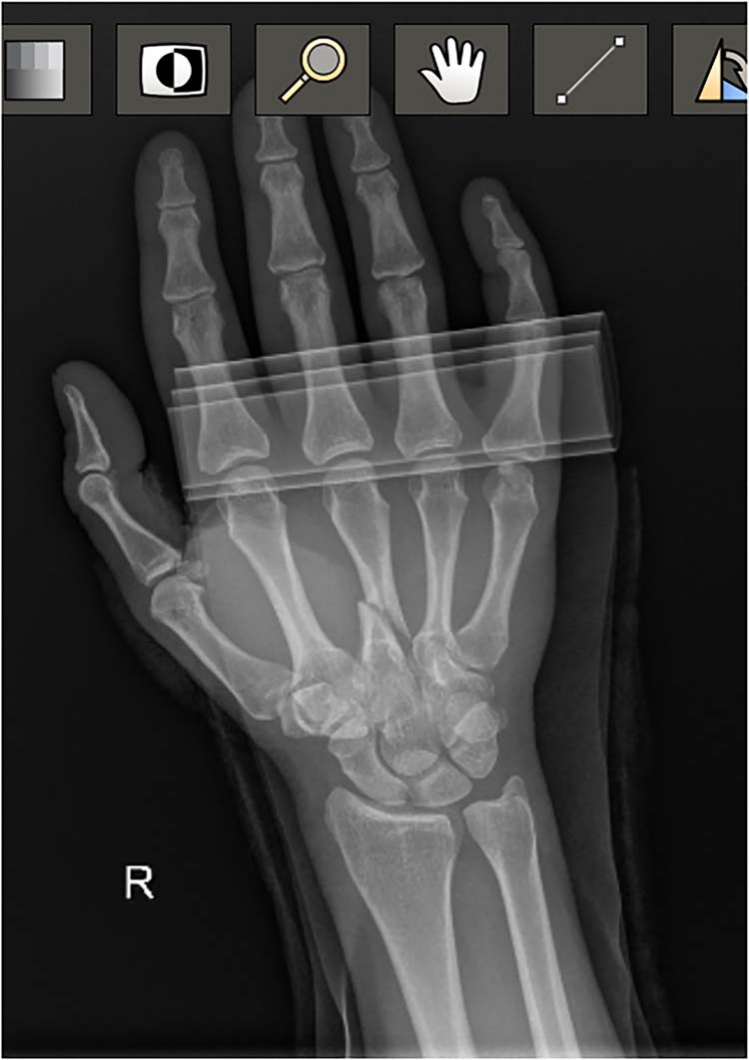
Diagnostic Radiograph 3.
